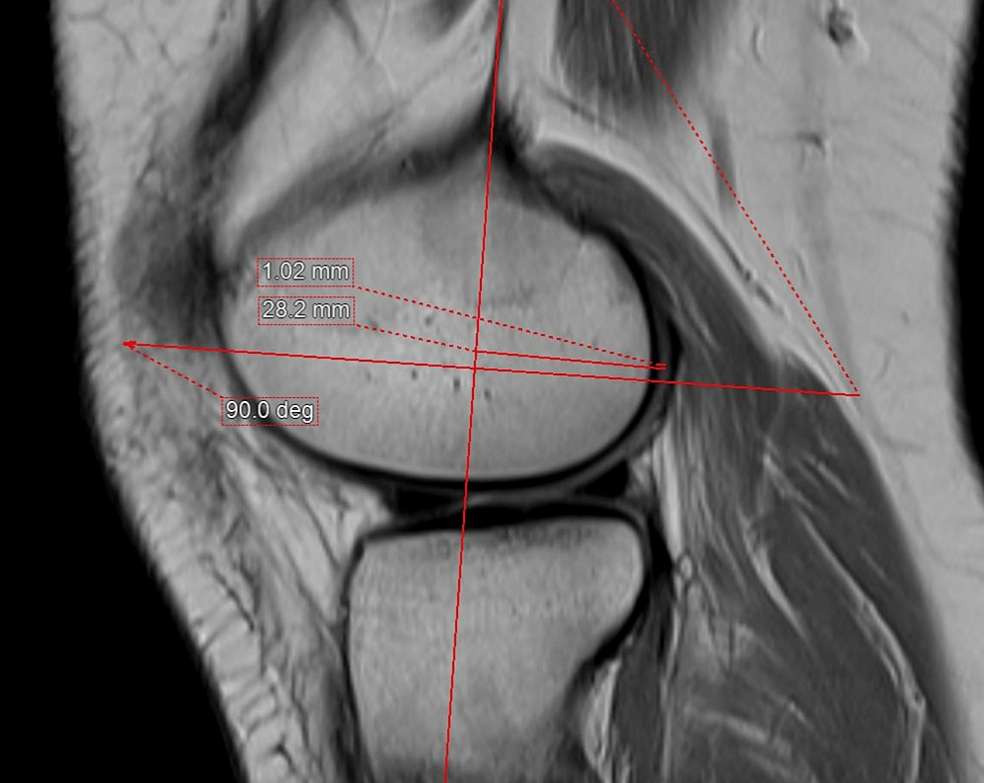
Sagittal proton-density MRI of the knee at the deepest part of the lateral femoral condyle. The midsagittal axis is translated laterally to measure the posterior condylar offset and posterior condylar cartilage thickness at the deepest part of the posterior condyle, perpendicular to the drawn femoral posterior cortical axis.
